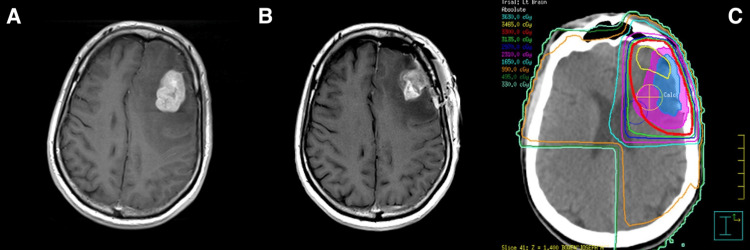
(A) MRI of brain metastasis prior to resection (B) MRI of tumor bed after resection and (C) 33 Gy/11 fractions dosimetry for a patient treated with conformal partial brain radiation
